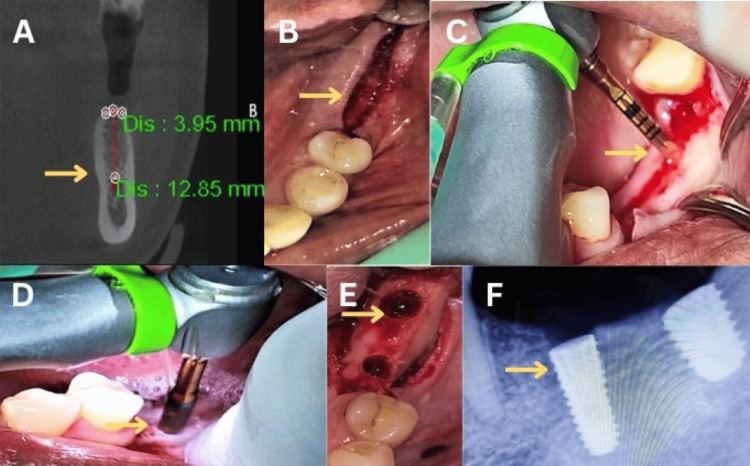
Osseodensification technique (A) Pre-operative cone-beam computed tomographic measurements of the mandible at the lower left first molar region (yellow arrow). (B) Mucoperiosteal incision in the alveolar crest (yellow arrow). (C) Pilot drilling (yellow arrow). (D) Densah bur drilling (yellow arrow). (E) Bur hole for implant placement (yellow arrow). (F) Post-implant intraoral periapical radiograph of lower left first and second molars (yellow arrow). This figure represents intraoral pictures of a patient from the study, and was published with the patient's consent.
